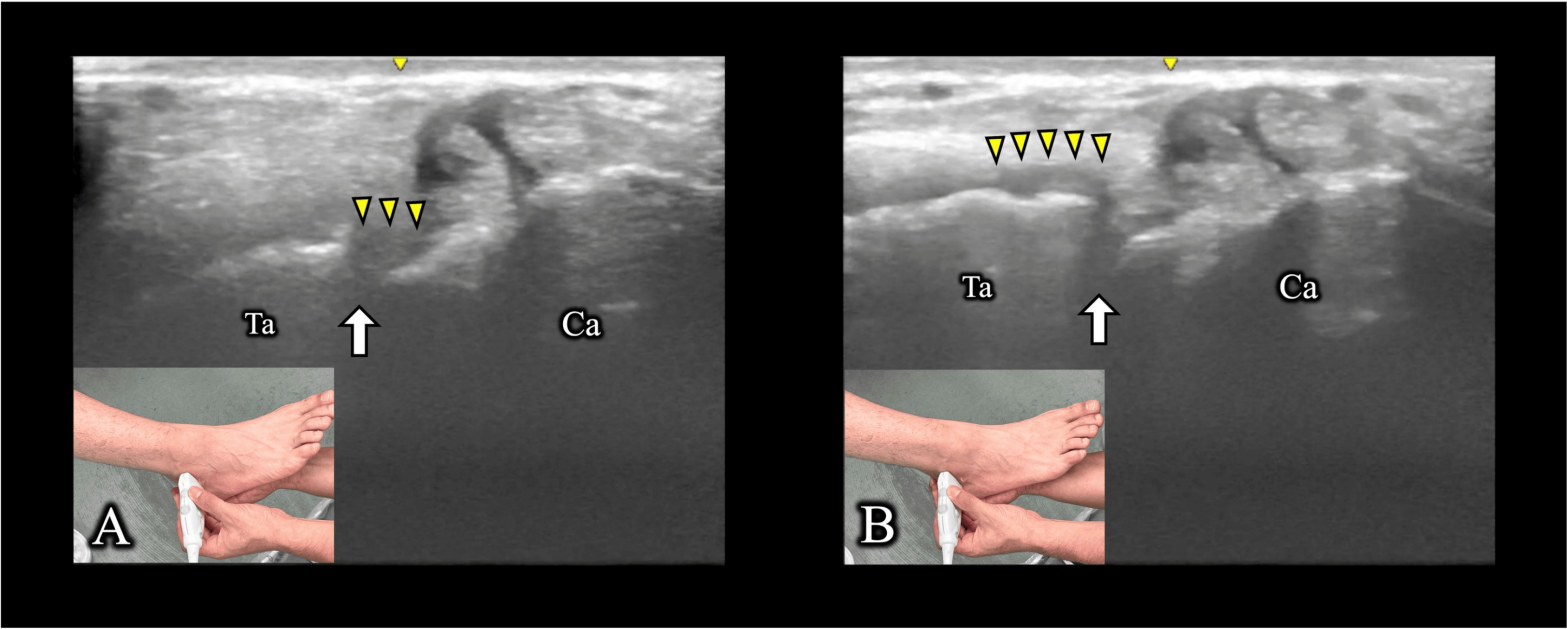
Ultrasound-guided forced pronation maneuver of the subtalar joint in maximal ankle plantar flexion (A) Starting position (maximal ankle plantarflexion, subtalar joint supination); (B) During forced pronation (maximal ankle plantarflexion, subtalar joint pronation). Arrows indicate the subtalar joint; arrowheads indicate hypoechoic areas. Owing to the narrowing of the joint space of the subtalar joint, the hypoechoic area, which is thought to be edema, moved outside the joint.
